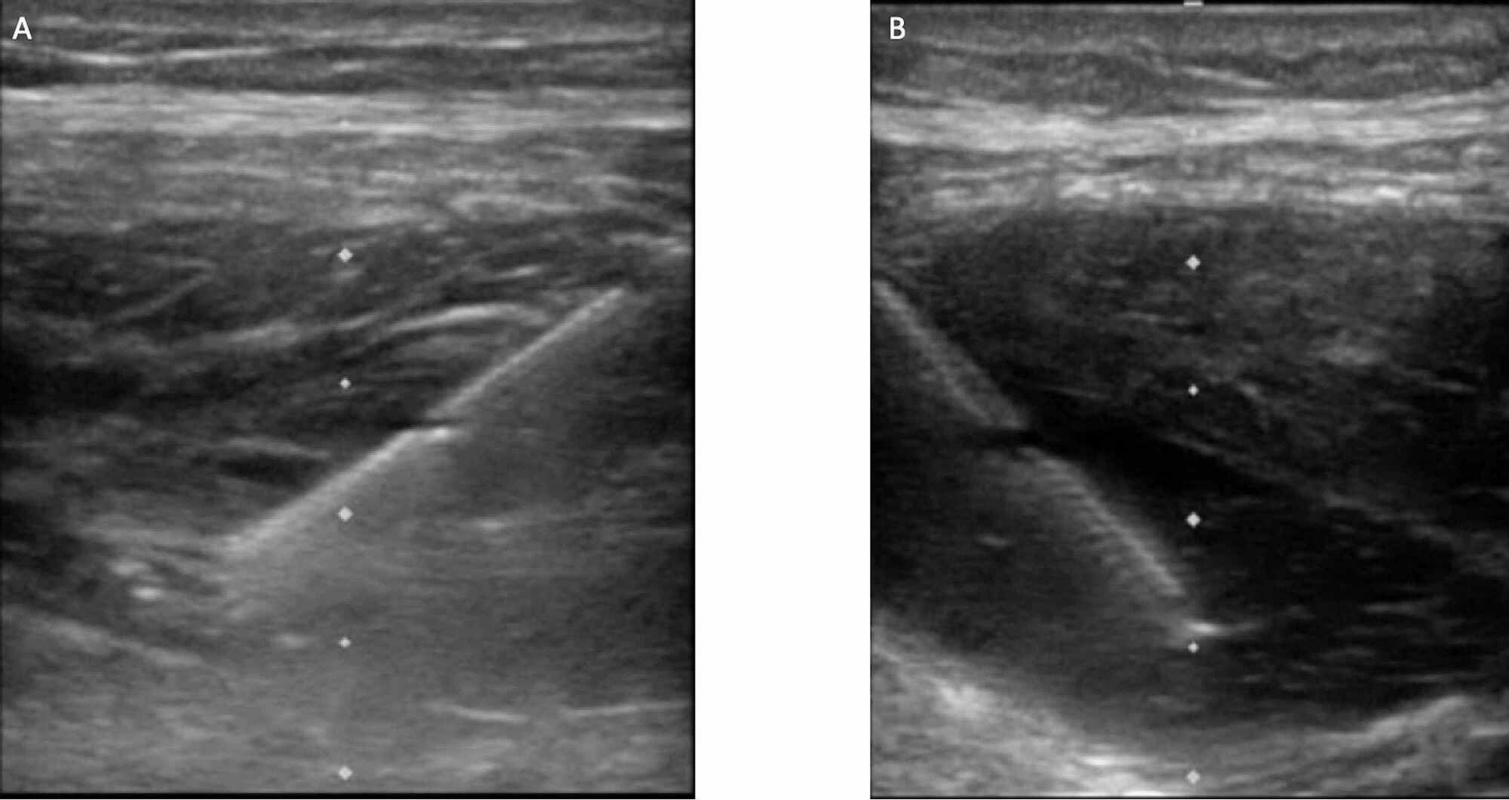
Ultrasound guided deposition of local anesthetic in the right (A) and left (B) transversus abdominal plane (TAP) to block somatic sensibility of the anterolateral abdominal wall (T7 to L1)
